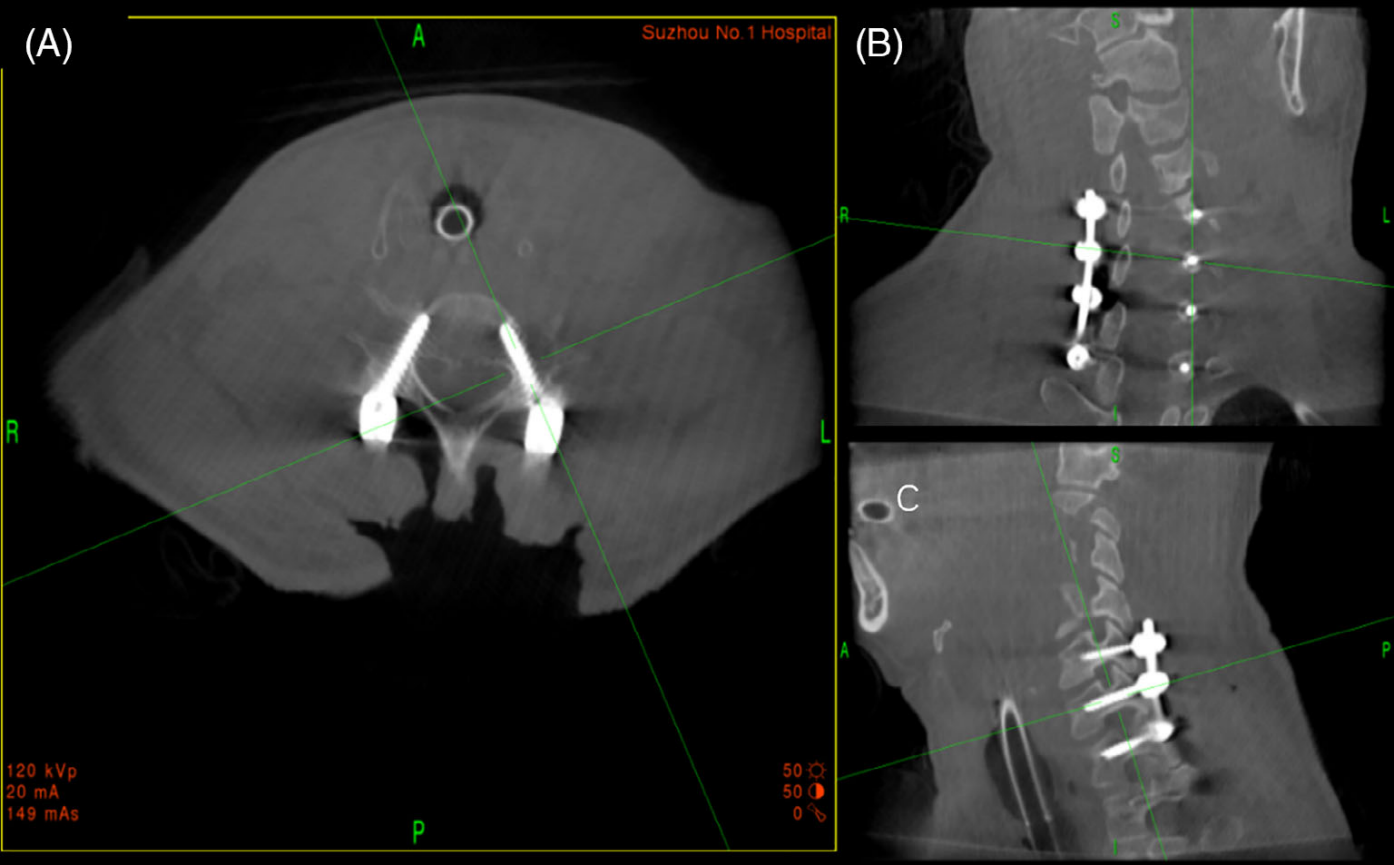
Intraoperative 3D image data obtained by O‐arm showing cervical pedicle screw (CPS)s were accurately inserted in the cervical pedicle. (A) Intraoperative cross‐sectional CT of cervical spine after CPS insertion. (B) Intraoperative cervical spine coronal CT after CPS insertion. (C) Intraoperative cervical spine sagittal CT after CPS placement.
